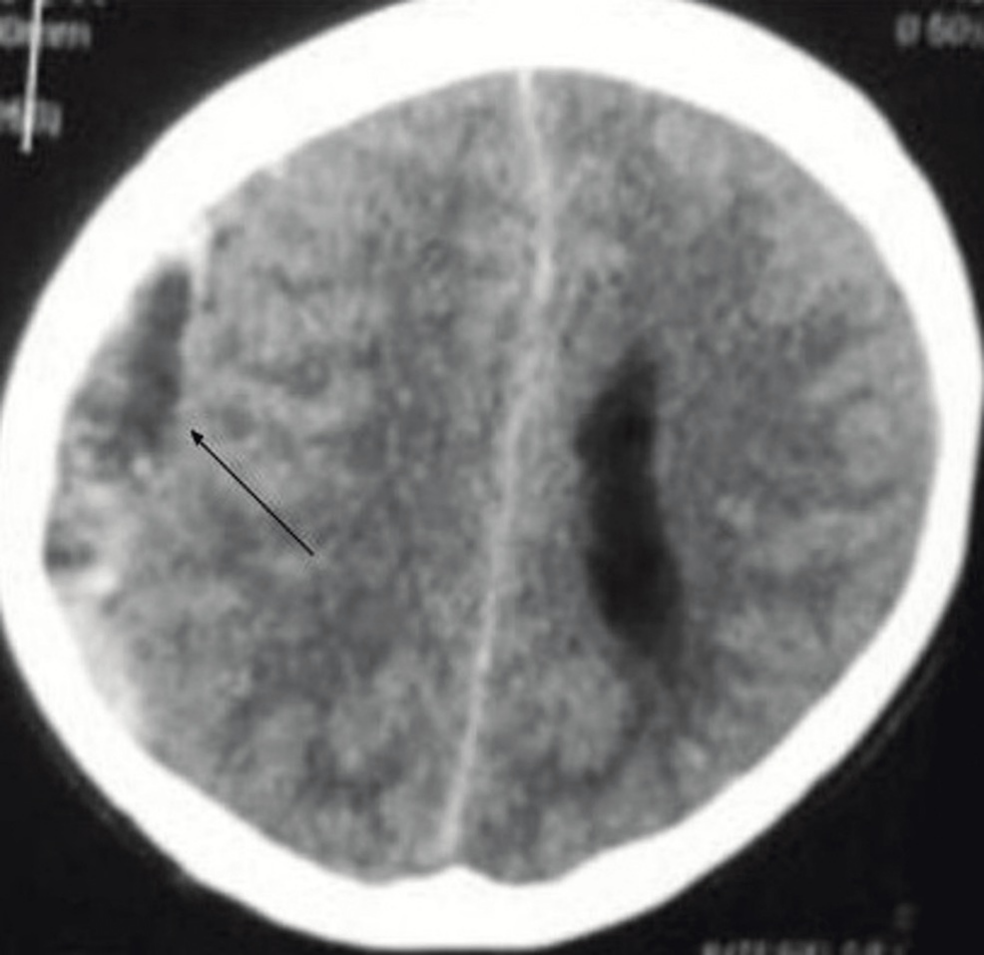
A CT scan of the brain with axial view showing a hypodense crescent-shaped lesion in the fronto-parietal region (arrow)
